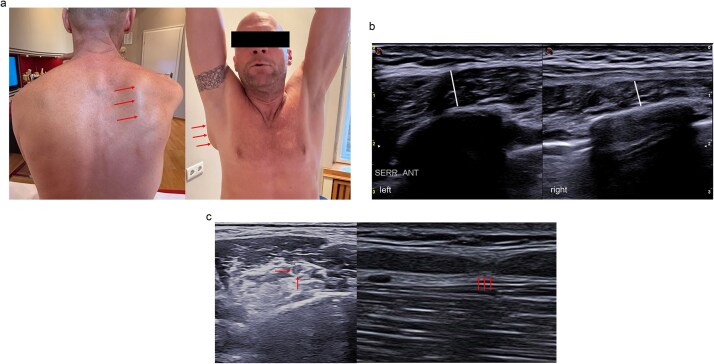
(a) Clinical image of right scapula alata with elevation of the medial scapular border (marked with arrows). (b) Sonography showing slight atrophy of the right serratus anterior muscle. (c) High-resolution ultrasound showing perifocal thickening in the axial view (arrows) and an hourglass-shaped nerve constriction (arrows) in the longitudinal view of the long thoracic nerve.
